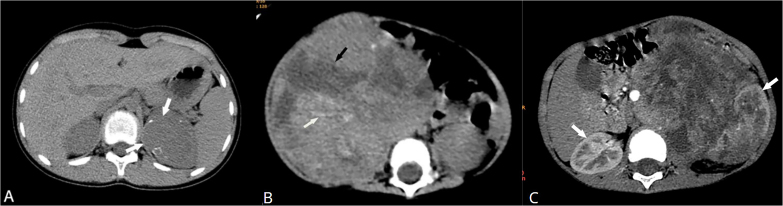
Representative CT images of pediatric renal tumors: A – clear cell carcinoma, B – malignant rhabdoid tumor, C – clear cell sarcoma. Images adapted from Zhu et al.^
